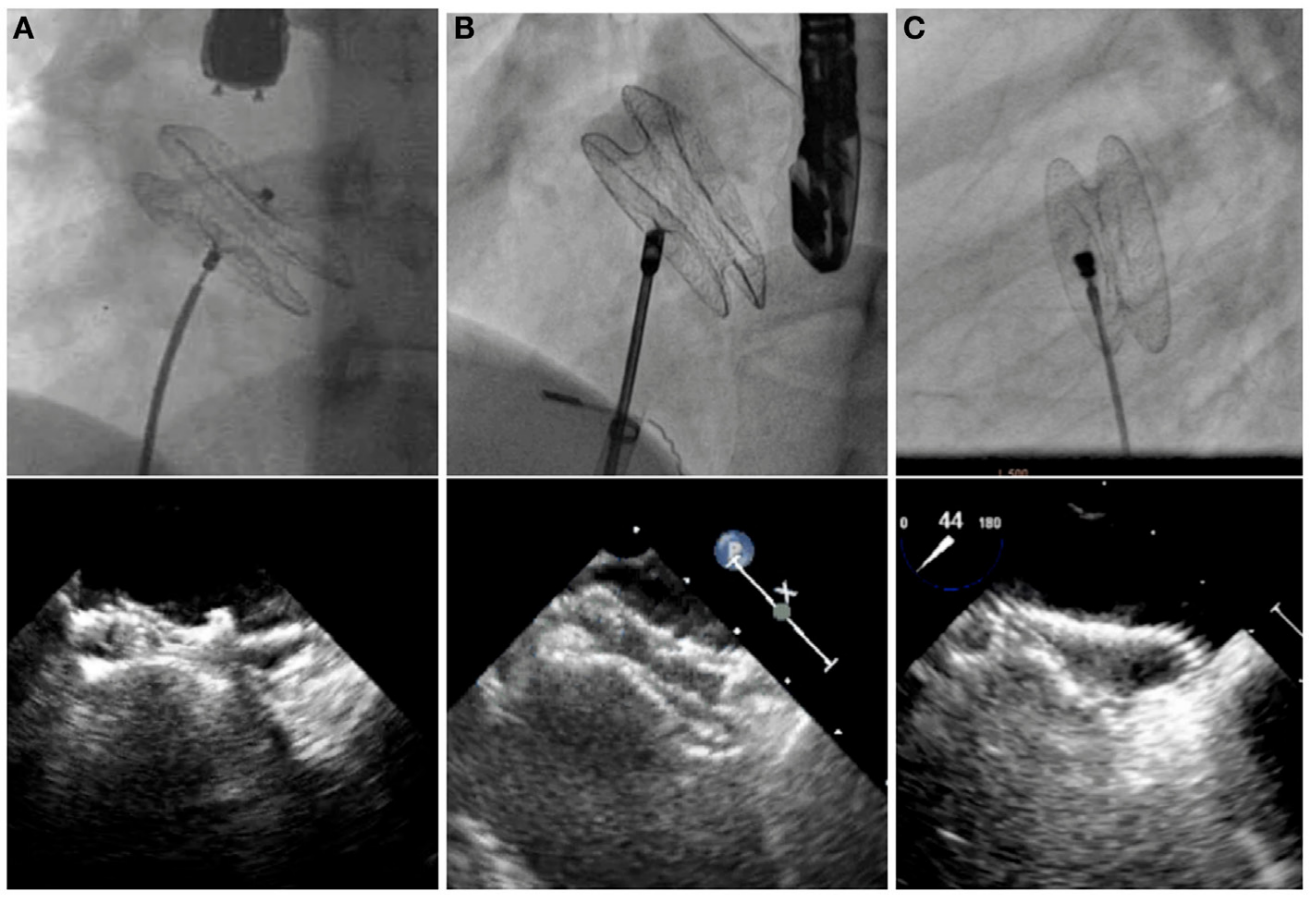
Fluoroscopic and echocardiographic pictures of various ASD devices. Amplatzer Septal Occluder (A), Occlutech Figulla Flex II Septal Occluder (B), and CeraFlex ASD Occluder (C).
